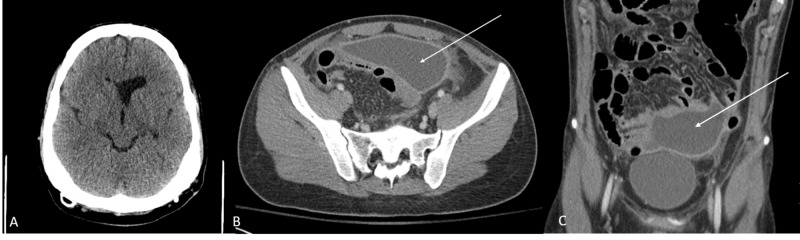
CT without contrast of head and abdomen CT head without contrast (A) showing stable ventricular size on admission. CT abdomen without contrast showing abdominal pseudocyst (arrow) on axial (B) and coronal (C) sequences.
